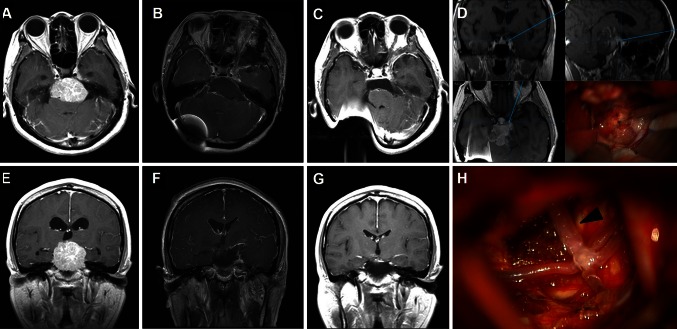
a and e Preoperative MR images of petroclival meningioma in a 45-year-old female patient who underwent ventriculoperitoneal shunt placement before tumor removal. b and f Immediate postoperative MR images show that the tumor was totally removed via the MLSO approach. c and g MR images show no recurrence of tumor 6 months after surgery. d The petroclival junction lesion and the tumor. h The basilar artery (*black arrow head*) was freely exposed after tumor resection
